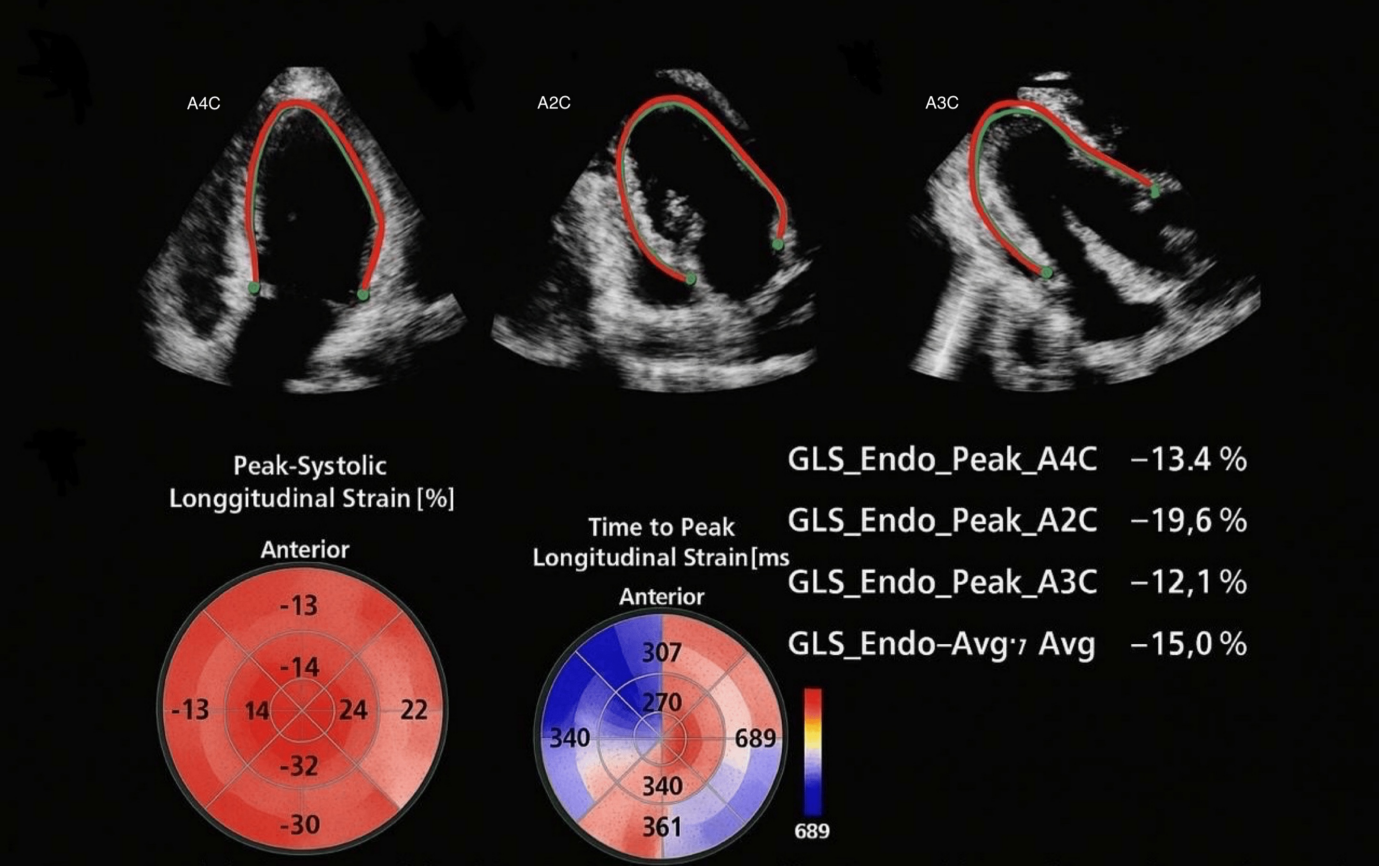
Global longitudinal strain (GLS) assessed by 2D tracking echocardiography. A4C: apical four-chamber view; A2C: apical two-chamber view; A3C: apical three-chamber view.
